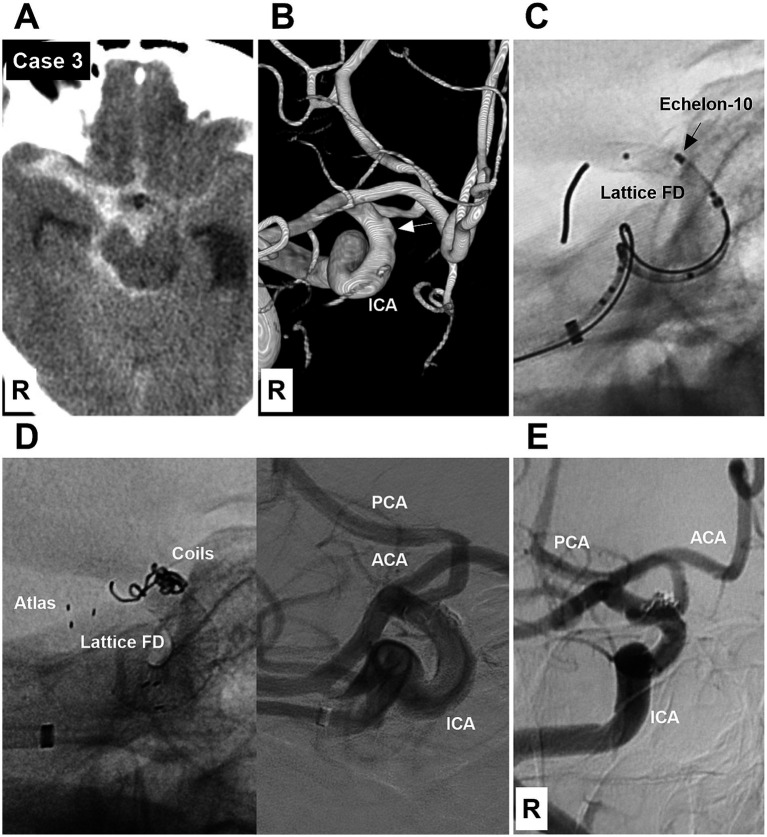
Case 3: The patient was treated by both coiling and deploying a Lattice FD device and a Neuroform Atlas stent (Type 2 EVT). (A) Computed tomography showing subarachnoid hemorrhage focused on the right suprasellar cistern. (B) DSA showing a Type I BBA (arrow) of the right supraclinoid ICA. (C) X-ray image showing an Echelon-10 microcatheter tip (arrow) at the BBA neck and a partially deployed Lattice FD device beyond the BBA neck. (D) Left panel: X-ray image showing that the BBA was embolized, and the Neuroform Atlas stent was used to fix the coil loop that escaped from the BBA due to the foreshortening of the Lattice FD device after deployment. Right panel: Postoperative DSA showing the patency of the supraclinoid vessels. (E) Six-month follow-up DSA showing that the BBA was cured, and the supraclinoid ICA was repaired. ACA, anterior cerebral artery; BBA, blood blister-like aneurysm; DSA, digital subtraction angiography; EVT, endovascular treatment; FD, flow diverter; ICA, internal carotid artery; PCA, posterior cerebral artery; R, right.
